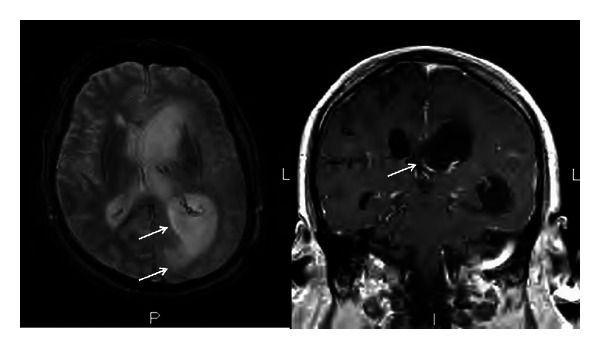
Brain MRI. Ventriculomegaly, increased halo surrounding the lateral ventricles, and debris within the ventricles with restricted diffusion and ependymal and subependymal enhancement (arrows).
